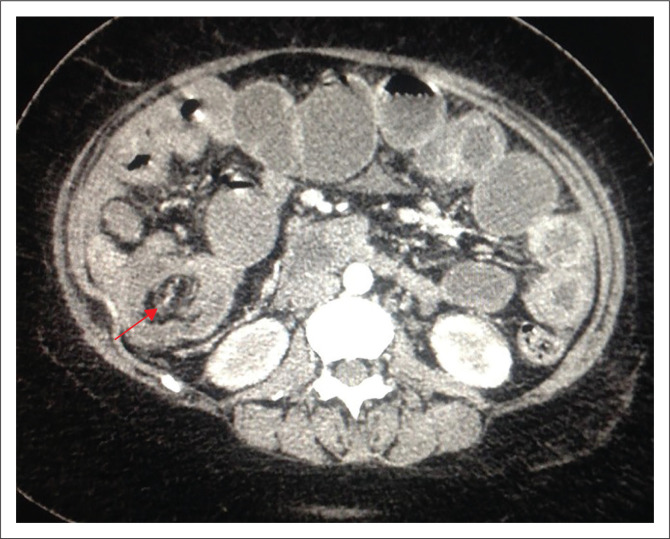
The patient’s computed tomography (CT) image (axial view) in the arterial phase. The red arrow indicates a target lesion at the ileo-caecal junction. Dilated loops of small bowel are seen.
